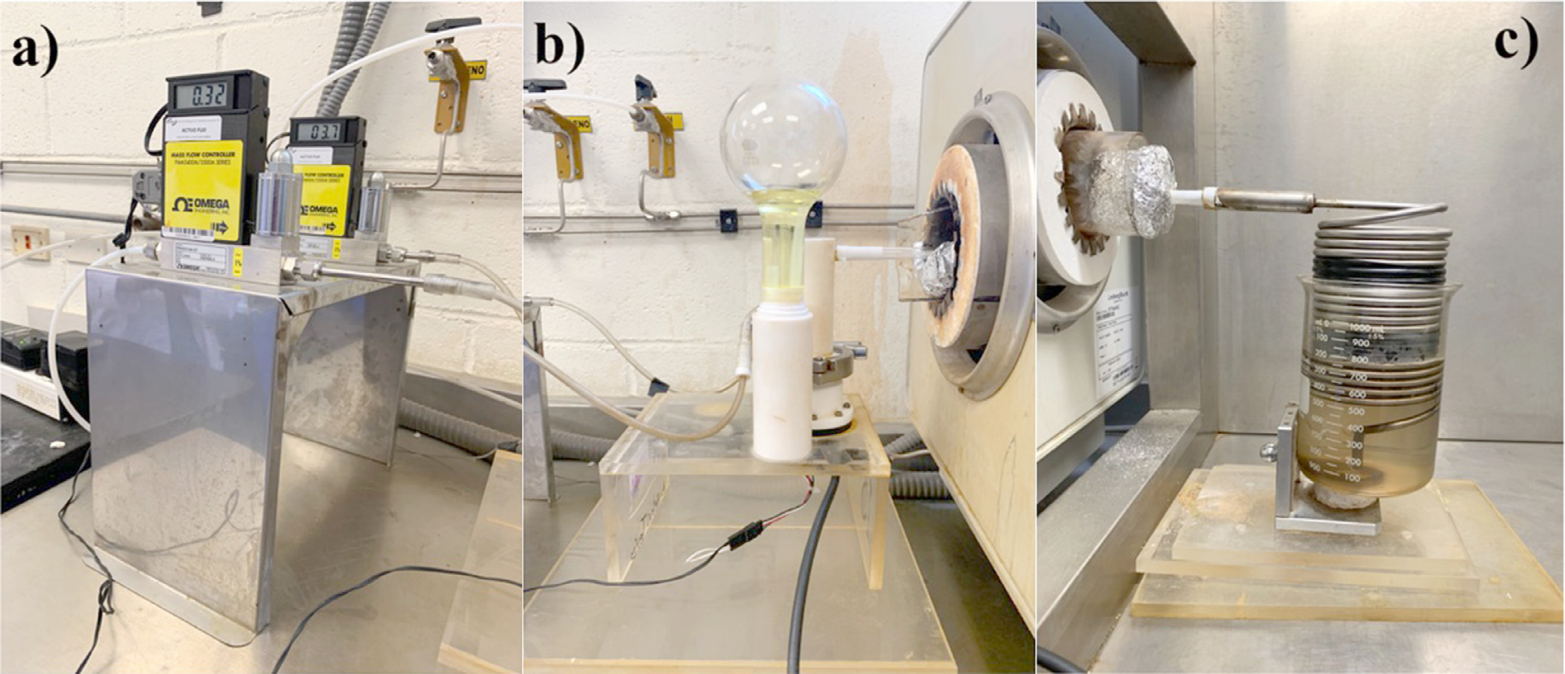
The fundamental components of the actual AACVD system. (a) The mass flow regulators. (b) The self-fabricated ultrasonic nebulizer and the precursor solution container are already assembled with the tubular reactor inserted inside the furnace. (c) The furnace outlet shows the tubular reactor coupled with the collecting system.
